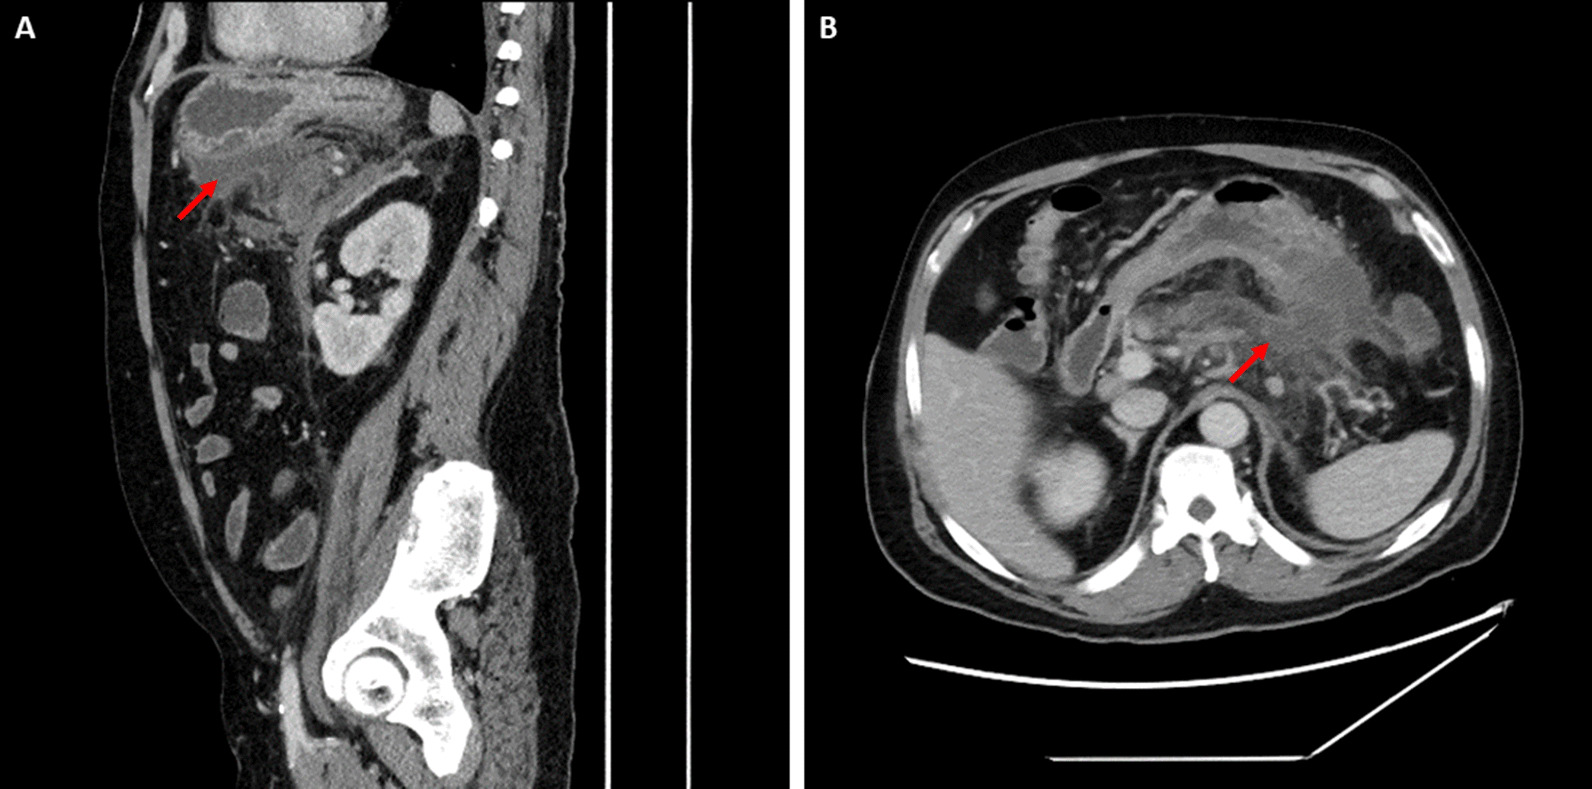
Computed tomography imaging of the abdomen and pelvis with intravenous contrast of a pancreatic pseudocyst on initial discovery (7.1 × 2.8 × 8.8 cm). A Sagittal view, B axial view. Red arrows denote pseudocyst
